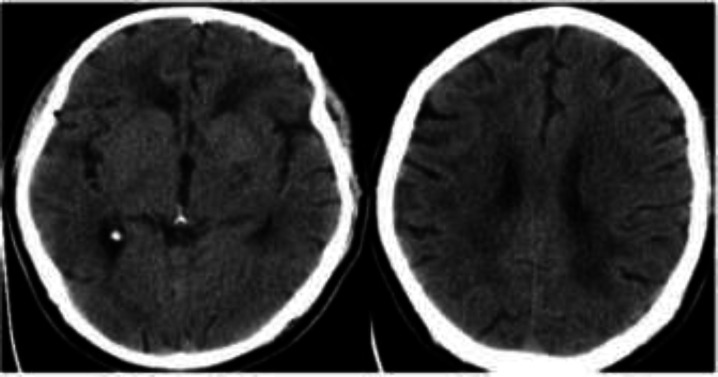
Ischemic cerebrovascular accident in the basal ganglia and thalamus of the left hemisphere in brain CT of case 4
